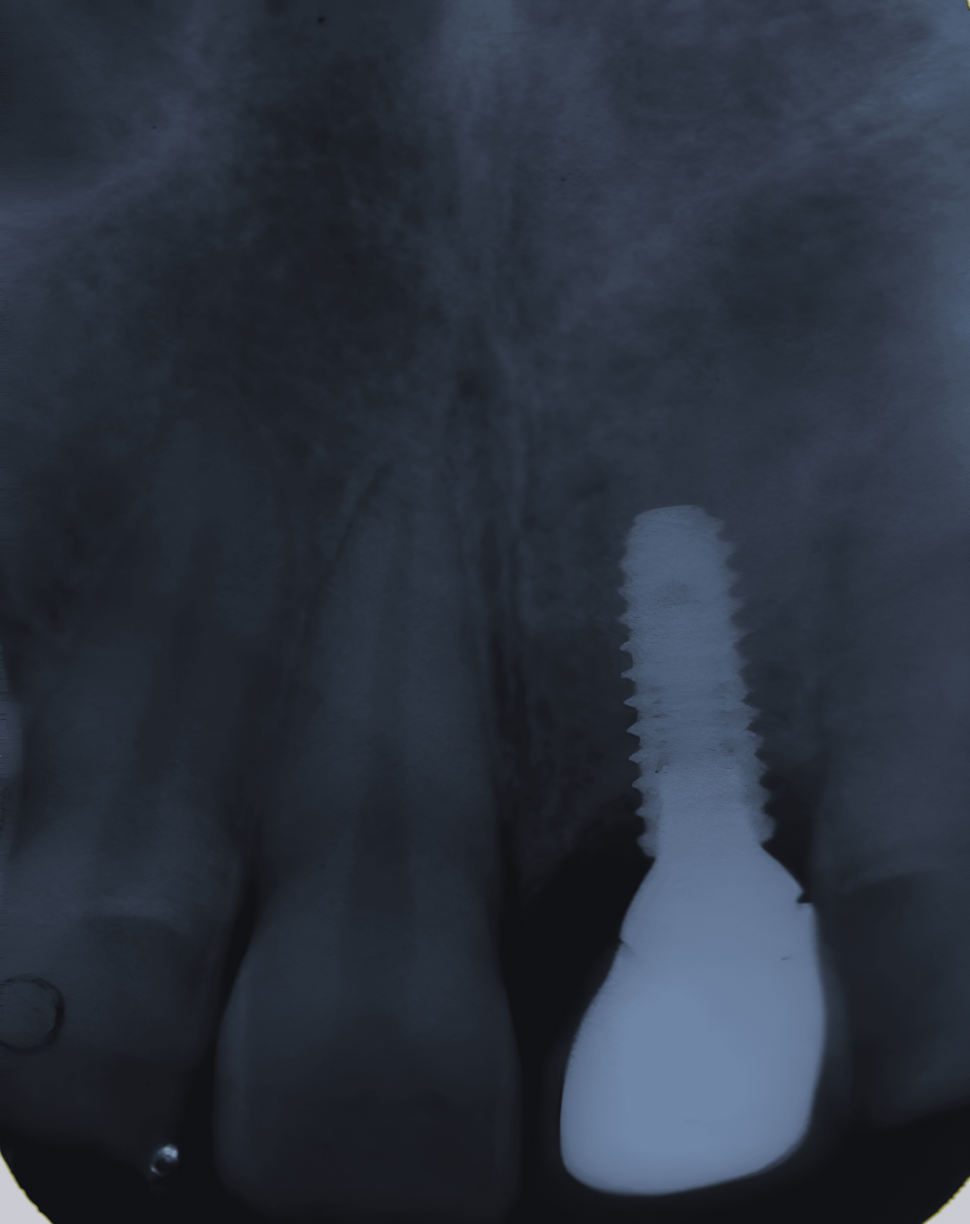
Case 1: Intra-oral periapical radiograph was taken for the patient at the follow-up visit after one year, showing mild bone loss of 0.2 mm, which is the expected amount of bone loss according to Albrektsson's criteria
